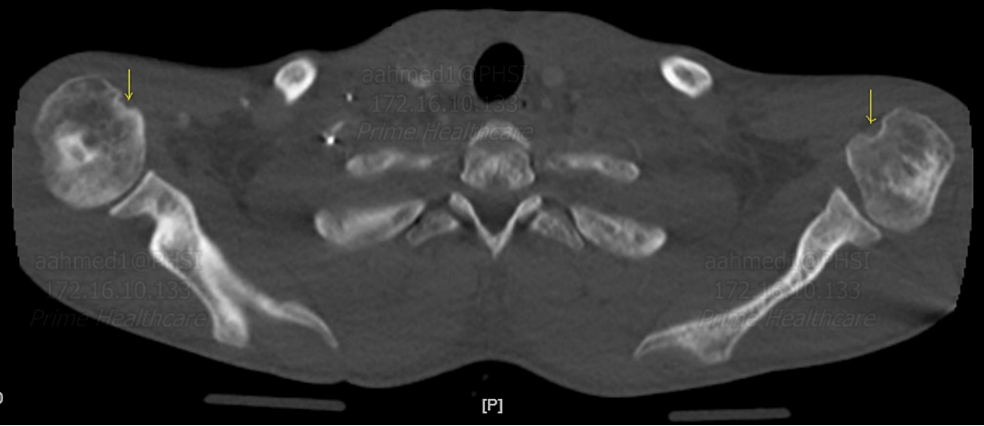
CTA chest axial bone window showing bilateral erosions and collapse of humeral heads consistent with avascular necrosis. CTA: CT angiography
